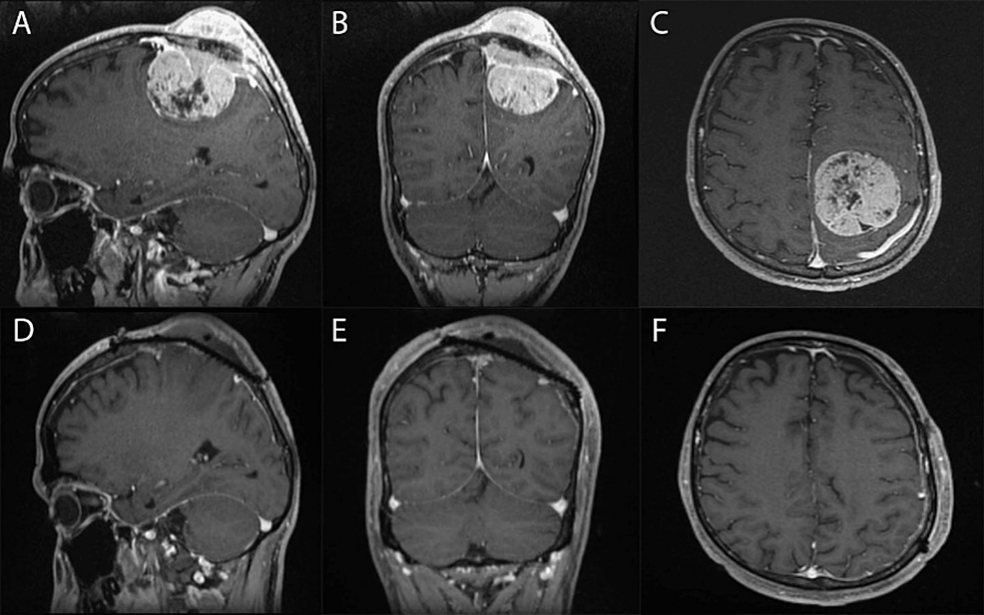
Pre and postoperative magnetic resonance imaging of the brain. A, B, and C: Preoperative sagittal, coronal, and axial magnetic resonance imaging (MRI) of the brain (T1 with contrast) showing a large parasagittal intra and extra-axial enchaining brain lesion in the left frontal lobe with an invasion of the superior sagittal sinus, skull, and scalp. D, E, and F: Postoperative sagittal, coronal, and axial MRI of the brain (T1 with contrast) showing gross total resection of the lesion with minimal residual over the superior sagittal sinus and skull defect covered by titanium mesh cranioplasty.
